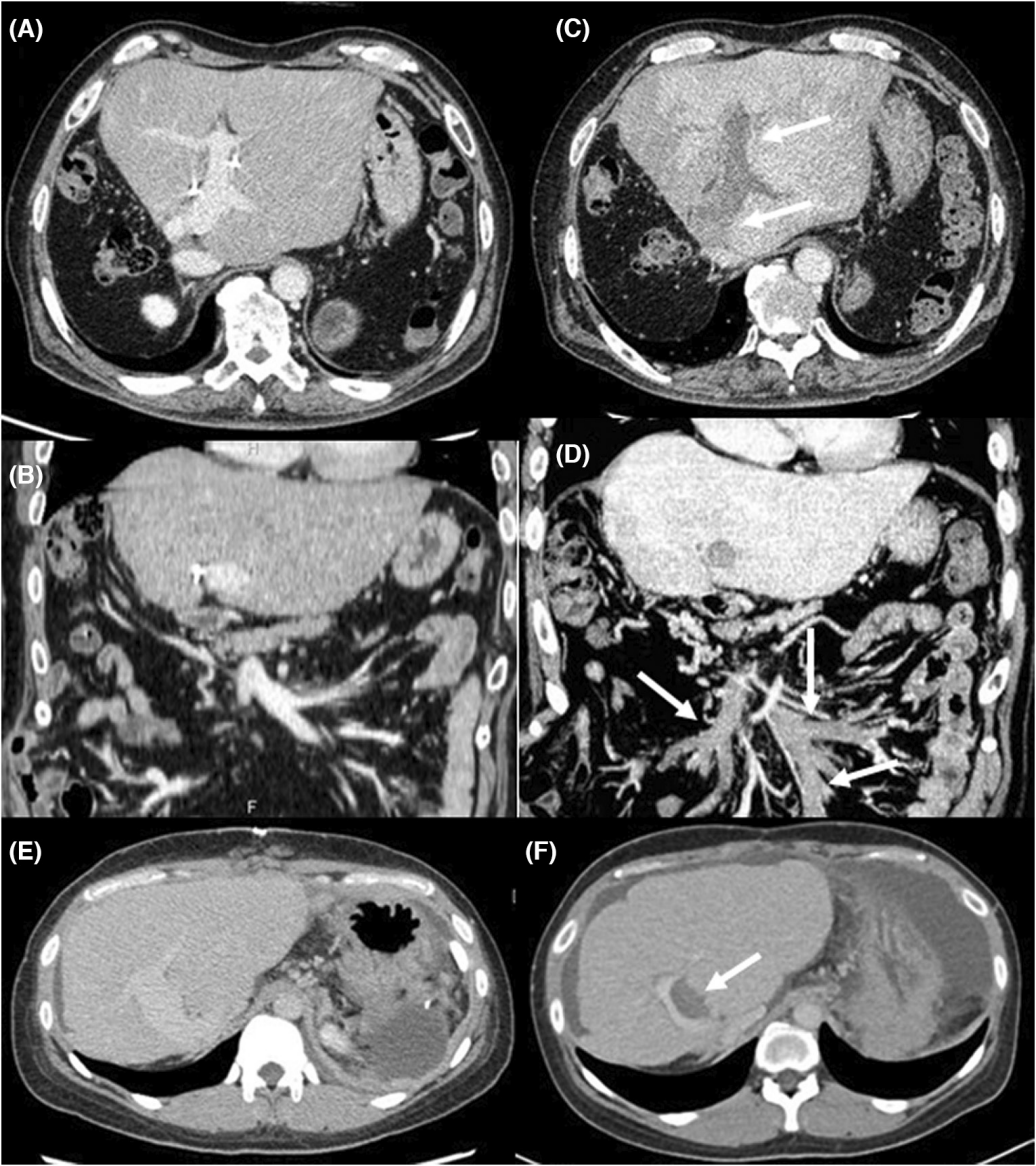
Enhanced abdominal computed tomography. Case 1 (A–D) and Case 2 (E,F). The portal vein was patent 1 year before the third vaccine dose in Case 1 (A and B) and before the fourth dose in Case 2 (E). However, the portal and superior mesenteric veins were completely obstructed by thrombosis 1 month after the third dose in Case 1 (C and D, arrows). Partial thrombosis in the portal vein was observed 2 weeks after the fourth dose in Case 2 (F, arrows).
